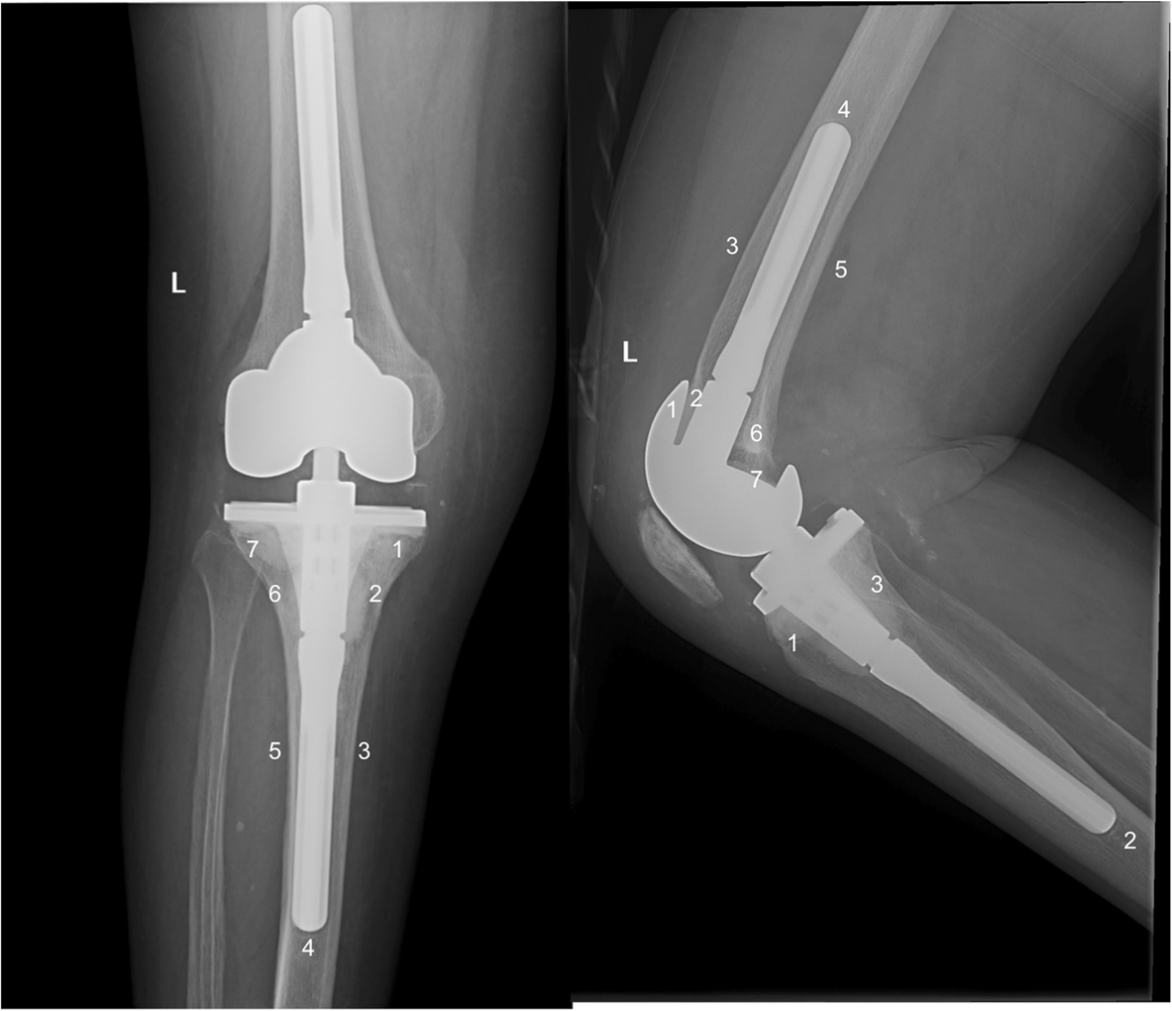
Radiographic zones for the evaluation of the radiolucent lines.
